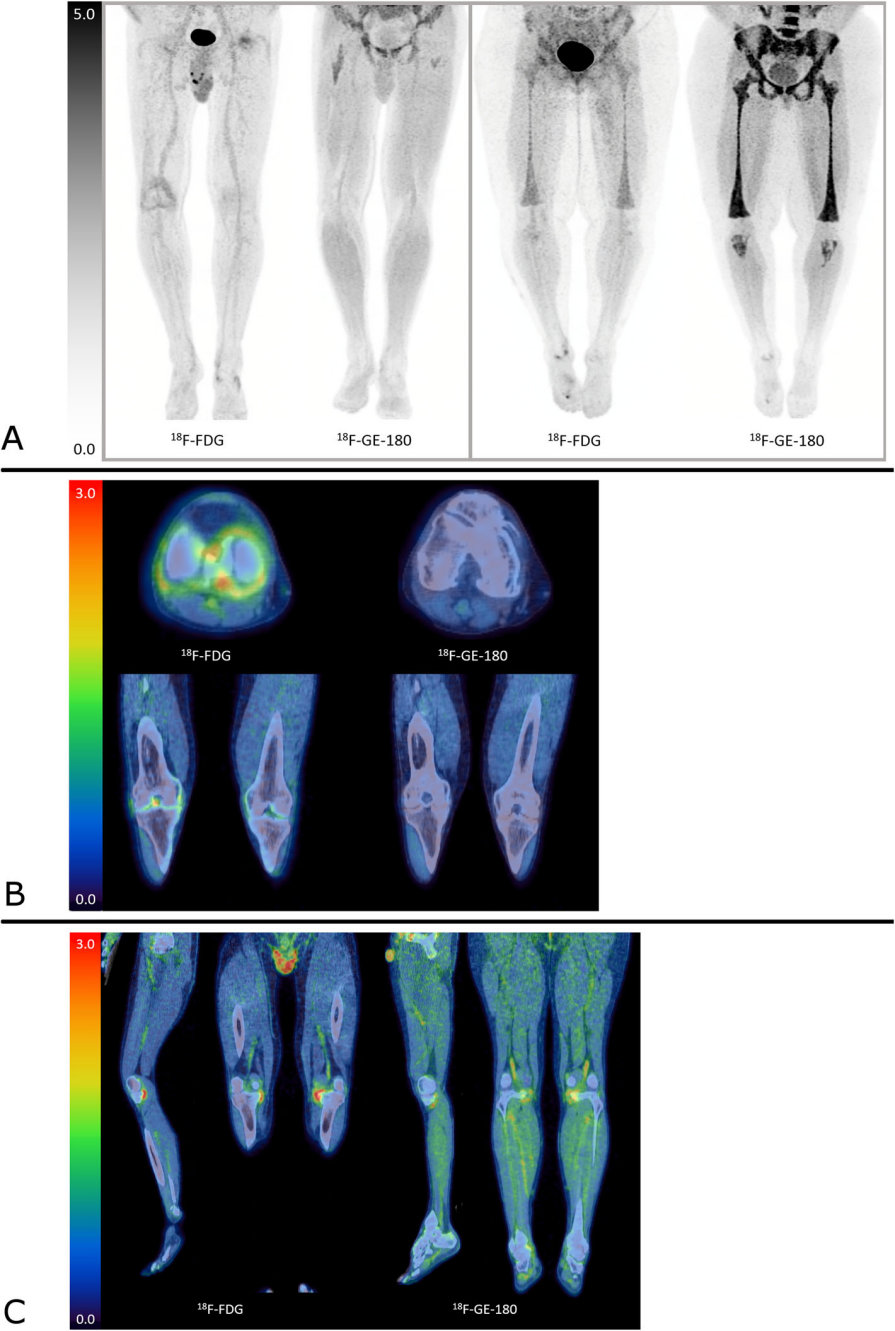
Representative imaging. a demonstrates maximum intensity projections (MIPs) of two patients imaged with ^18^F-FDG and ^18^F-GE-180. The two left images are of a patient who has increased right knee joint uptake with ^18^F-FDG, not seen with ^18^F-GE-180, which is also demonstrated on axial and coronal fused images in b. The images with ^18^F-GE-180 demonstrate a typical pattern of physiological uptake with marrow uptake in the proximal femurs and diffuse muscle uptake. The two right images of Fig. a are of a separate patient who demonstrates marked uptake within the marrow down to the proximal tibiae with ^18^F-GE-180. A similar but less intense uptake is seen with ^18^F-FDG and is presumed related to a reactive marrow. c are coronal and sagittal images of a patient with increased uptake in the medial aspects of both knee joints which is much more marked with ^18^F-FDG, compared to ^18^F-GE-180. The ^18^F-GE-180 images also demonstrate increased muscle and marrow uptake. The scale on all figures is SUV (g/mL)
